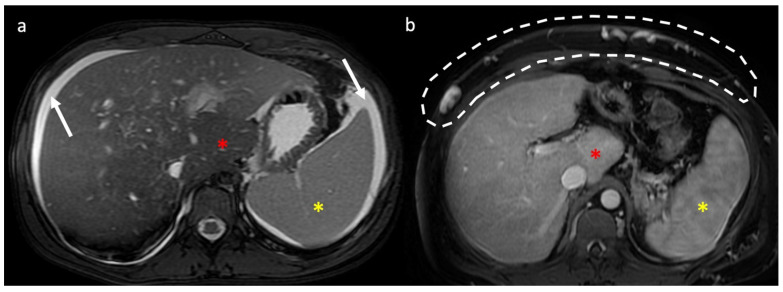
Axial T2 weighted (a) and portal venous phase (b) MR images show signs of portal hypertension including ascites (arrows), splenomegaly (yellow asterisk), and caput medusae (white circle). Notice the presence of caudate lobe enlargement (red asterisk) in both cases.
